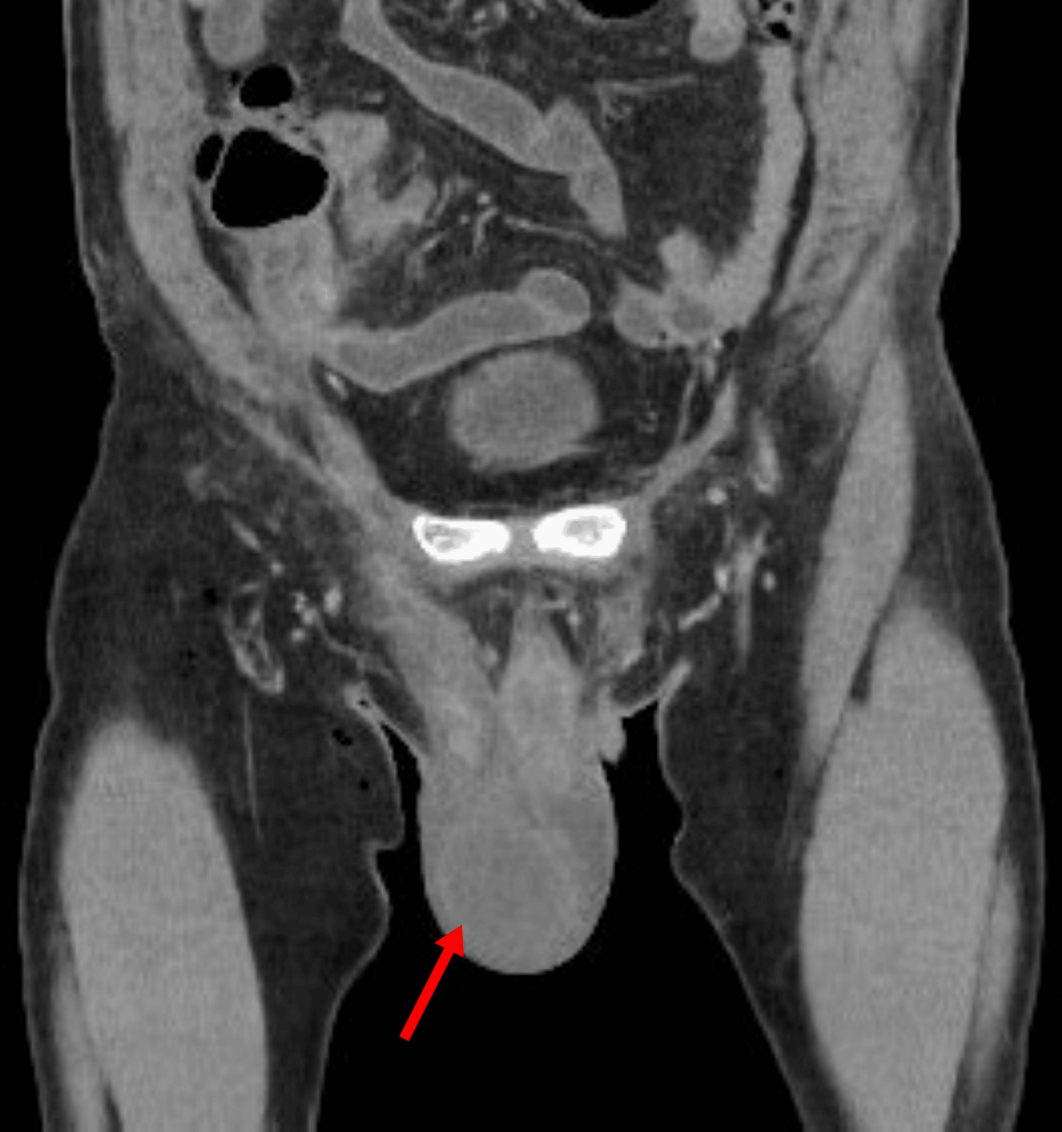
The CT scan shows findings of large right hydrocele labeled with an arrow.
